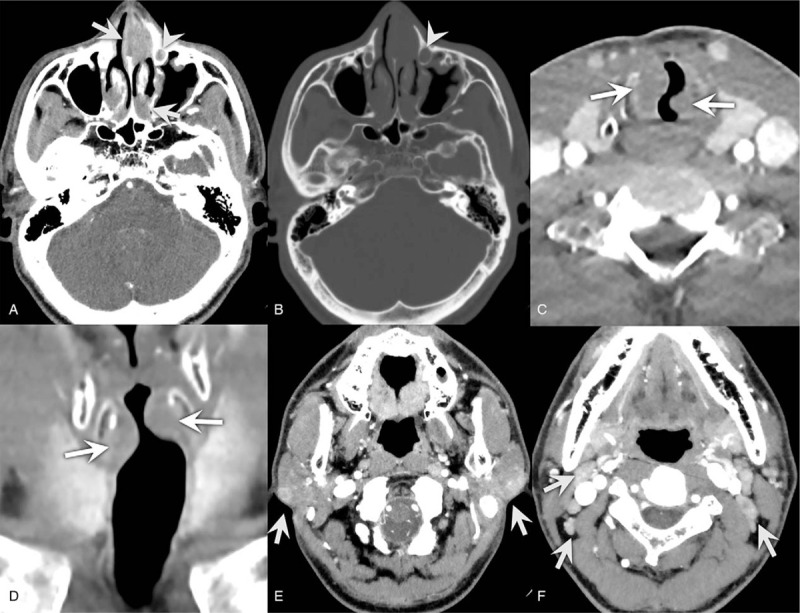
CT images of Rosai-Dorfman disease involving nasal cavities, subglottic larynx, and cervical lymph nodes. (A) Contrast enhanced CT image shows well-defined homogeneously enhancing soft tissue masses in both nasal cavities (arrows) and left nasolacrimal duct (arrowhead). (B) On bone setting image, left nasolacrimal canal is widened due to the nasolacrimal duct mass (arrowhead). (C, D) There are mildly enhancing soft tissue masses in both sides of the subglottic larynx, resulting in mild airway narrowing (arrows). (E, F) There are multiple enlarged lymph nodes showing homogeneous enhancement in both parotid glands and both level II (arrows).
